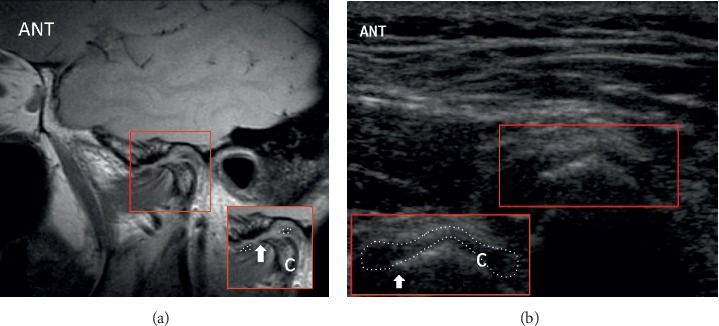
Osteoarthritis with the deformed articular surface of the condyle with a large anterior marginal osteophyte and deformed disc with central thinning on (a) MR and (b) ultrasound. A focal annotated view was provided to aid visualization with the articular disc outlined with a dotted contour and the osteophyte indicated by an arrow; anterior = “ANT”; condyle = “c.”
